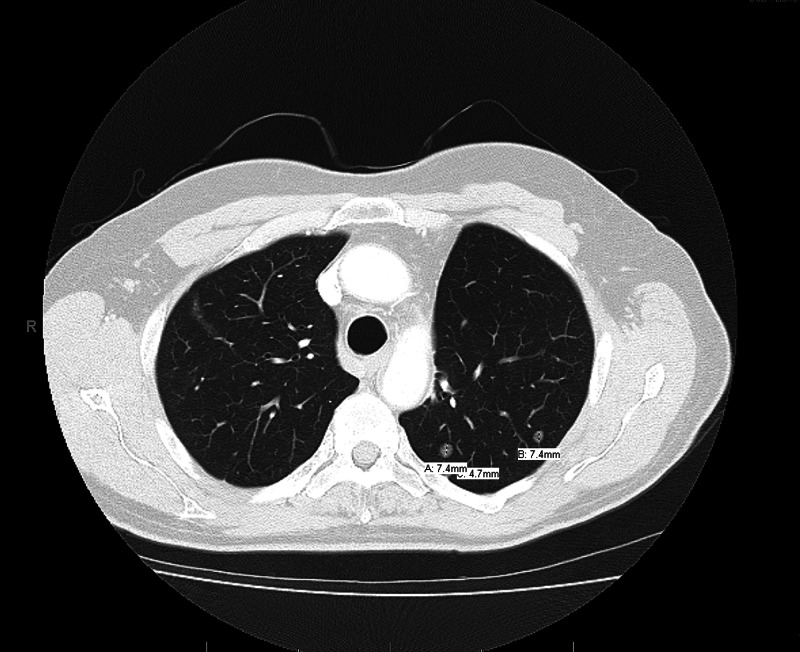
CT thorax revealing multiple pulmonary nodules with the largest being 7.4 mm CT: computed tomography
